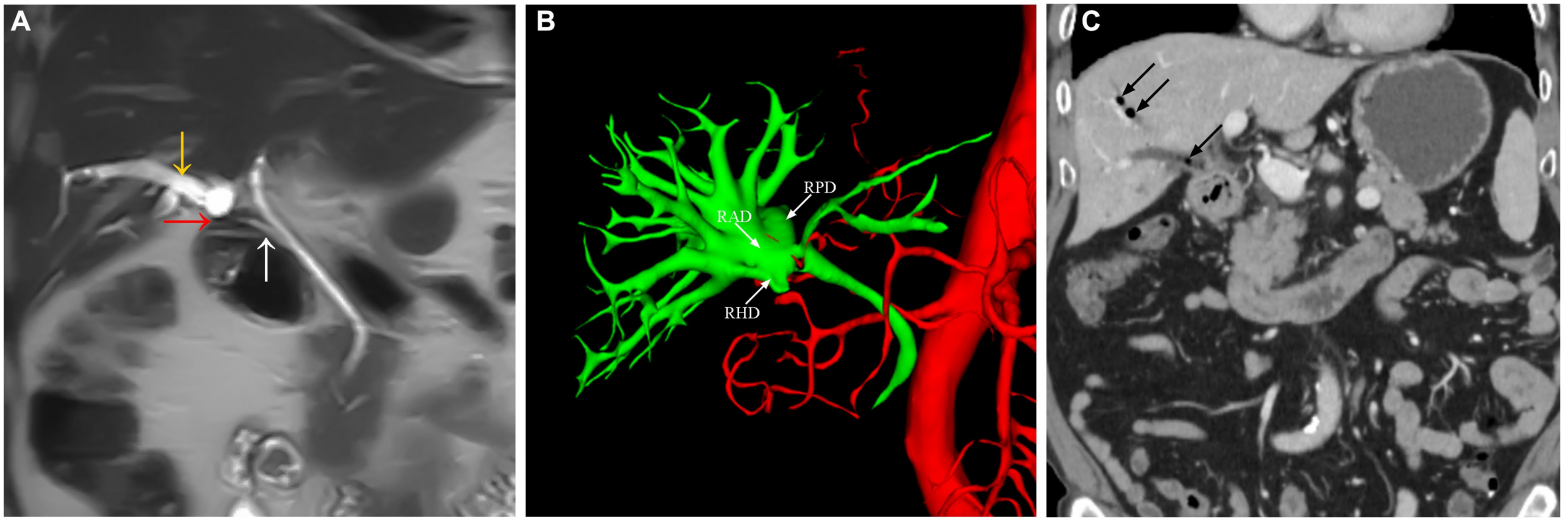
The abdominal MRI scan (A) showing the right hepatic bile duct (yellow arrow), a fistula between the right hepatic duct and the duodenal bulb (red arrow), and a low confluence of the right hepatic duct into the common bile duct (white arrow). Three-dimensional cholangiography (B) showing the right anterior hepatic duct, right posterior hepatic duct, right hepatic duct, and an abnormal opening with stenosis in the right hepatic duct. The abdominal CT scan (C) revealed the presence of gas within the right intrahepatic duct (black arrow).
